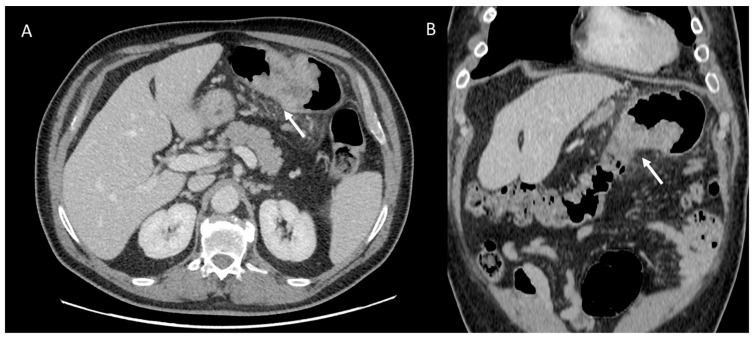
Man with transverse colon cancer (white arrows): in (A) (axial) and (B) (coronal) CT assessment.
